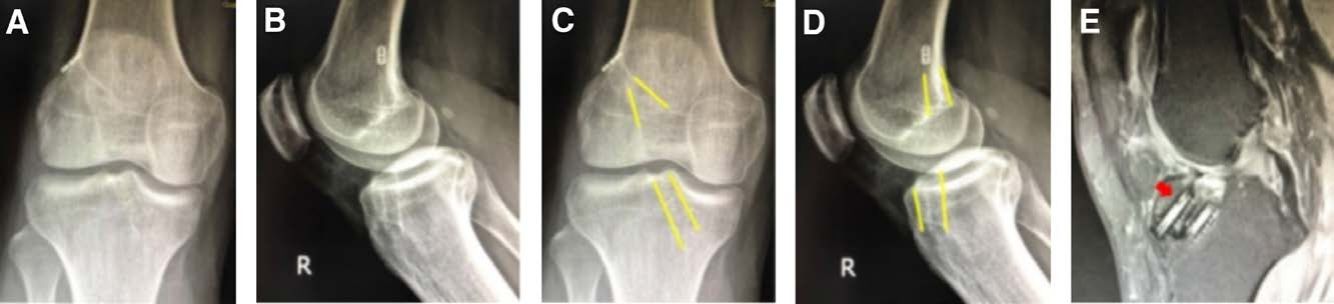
(A and B) DR: the tibial tunnel was in a good position; however, it appeared significantly anterior in the lateral view. (C and D) The yellow line marks the location of the femoral and tibial tunnels, with the tibial tunnel identified as being positioned anteriorly. (E) MRI image: at 4 months postoperatively, adjustment was made to the tibial tunnel position.
